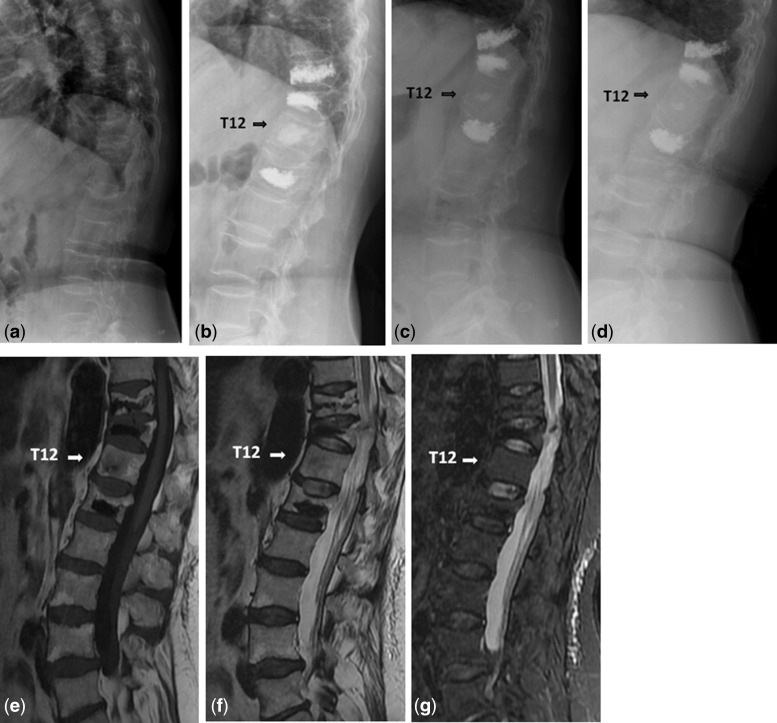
Images obtained in a 63-year-old woman with OVCFs. (a) The lumbar lateral radiograph before procedure. (b) T10, T11 and L2 were treated with vertebral cement augmentation, where T12 was a sandwich vertebra. (c) Follow-up after 12 months showed no loss of T12 height. (d) Follow-up after 22 months showed no loss of T12 height. (e and f) T1- and T2-weighted images indicated low intense signal, indicating the calcium phosphate component which remains intact for years providing an osteoconductive matrix for new bone ingrowth. (g) Image showed no high signal intensity
